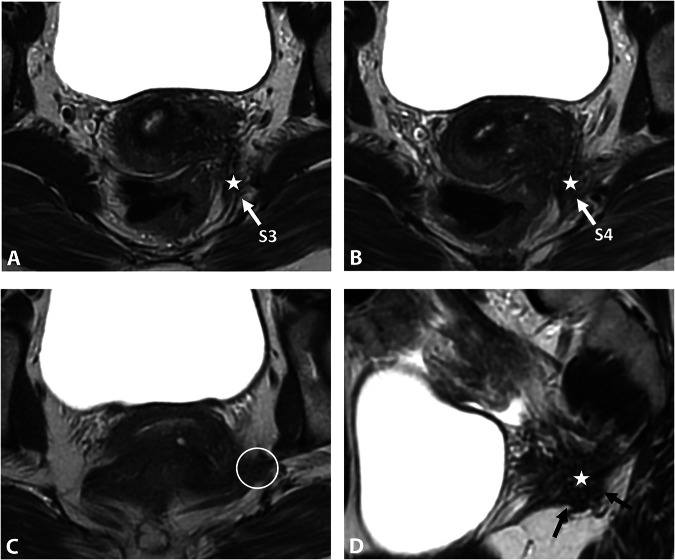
DE in a 29-year-old woman with deep dyspareunia and left pudendalgia. A–C Axial and (D) sagittal T2W MR images show fibrotic nodular infiltration of the left posterolateral parametrium (stars) extending to the pelvic wall and to the iliococcygeus muscle of the levator ani muscle (D, black arrows), involving the left inferior hypogastric plexus, the left sacral nerves courses S3 and S4, and the level of origin of the left proximal pudendal nerve (C, circle). The patient was treated medically with hormone therapy
